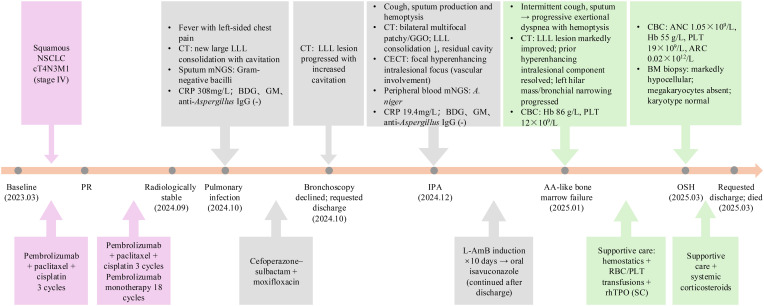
Timeline of events in this case. NSCLC, Non–small cell lung cancer; LLL, Left lower lobe; CT, Computed tomography; GGO, Ground-glass opacities; CECT, Contrast-enhanced computed tomography; mNGS, Metagenomic next-generation sequencing; A. niger, Aspergillus niger; CRP, C-reactive protein; BDG, Serum (1,3)-β-D-glucan assay; GM, Serum galactomannan assay; IPA, invasive pulmonary aspergillosis; AA, Aplastic anemia; L-AmB, Liposomal amphotericin B; CBC, Complete blood count; Hb, Hemoglobin; PLT, Platelet count; RBC, Red blood cells; ANC, Absolute neutrophil count; ARC, Absolute reticulocyte count; BM, Bone marrow; rhTPO, Recombinant human thrombopoietin; SC, Subcutaneous; OSH, Outside hospital. Data from OSH were obtained from medical records provided by the patient.
